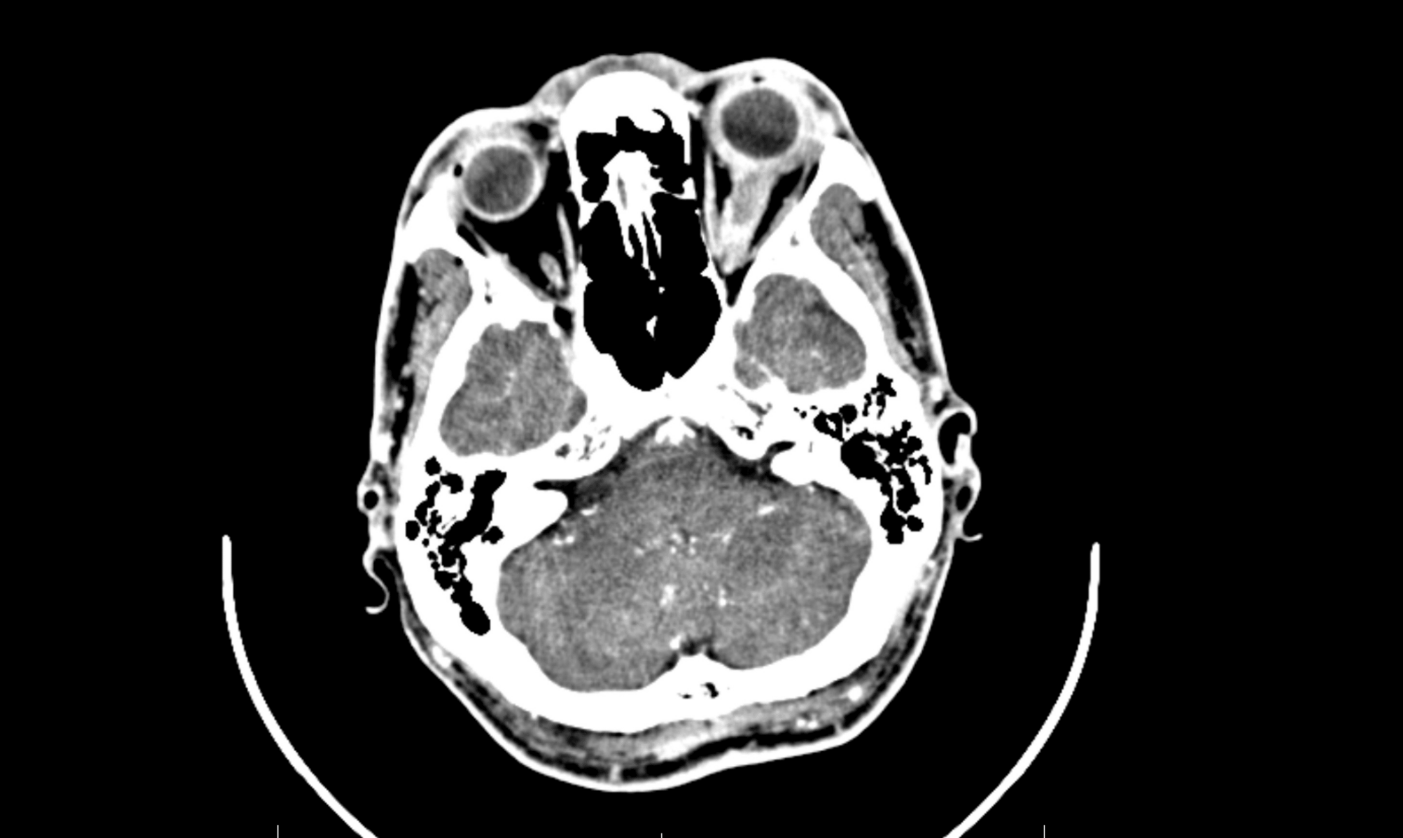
Axial contrast-enhanced computed tomography (CT) scan showing proptosis and an enlarged, enhancing optic nerve.
